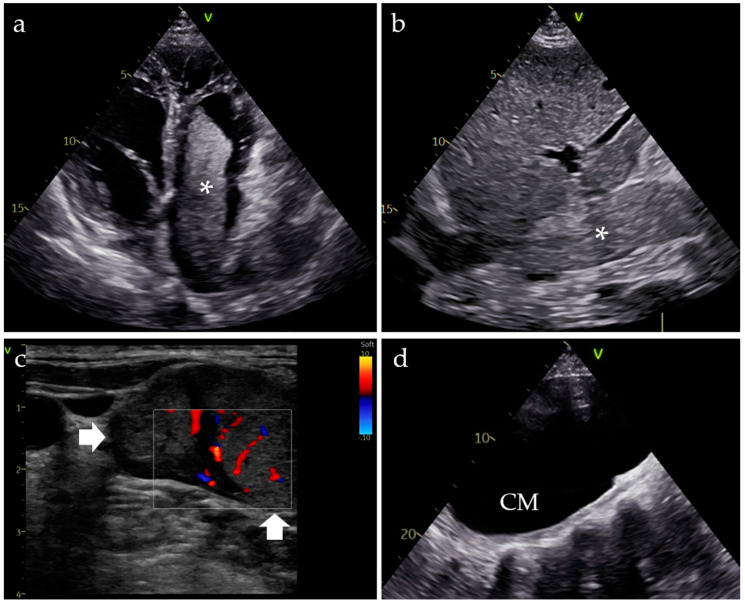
(a) Hyperechoic mass protruding from the right atrium into the right ventricle in diastole (*). (b) Thrombotic mass occupying almost the entire lumen of the inferior vena cava (*). (c) Enlarged lymph node in the supraclavicular fossa, with enhanced Doppler signal (arrows). (d) Large cystic mass (CM) in the lower pole of the left kidney.
